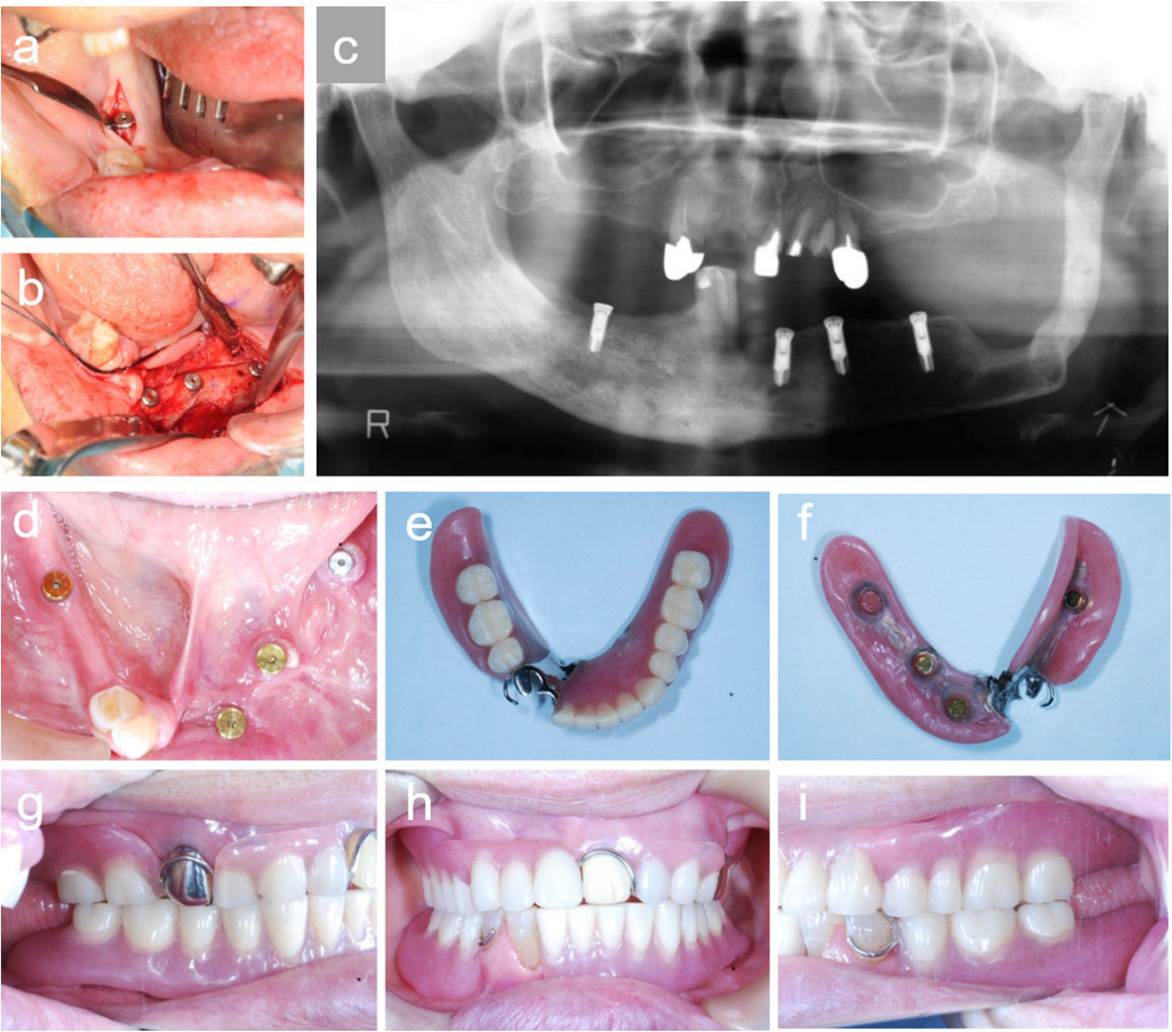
a, b Four dental implants were inserted into the distracted fibula. c Orthopantomogram after implantation. d–i Intraoral view after treatment: the implant denture was placed on the mandible
